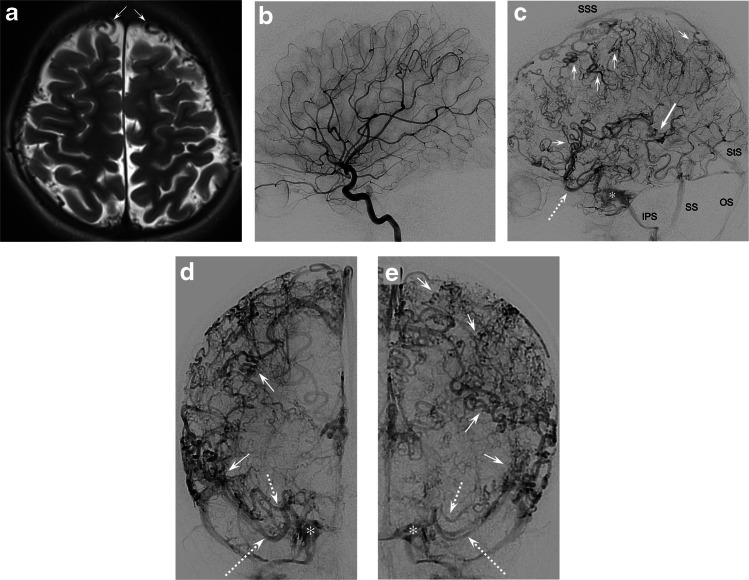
Non-restrictive cerebral venous dysgenesis in an 11-year-old girl with a history of infantile autism, attention deficit disorder, dyslexia, and camptodactyly. a Axial unenhanced T2-weighted image shows multiple small tortuous veins in the subarachnoid space above the cerebral hemispheres (*arrows*). b 2-dimensional (2-D) digital subtraction angiography (DSA), right internal carotid artery (ICA), mid-arterial phase, lateral: Normal appearance with no signs of arteriovenous shunting. c 2-D DSA, right ICA, mid-venous phase, lateral: filling of small superficial cortical veins with marked tortuosities and coilings over the right fronto-parietal and temporal cerebral hemisphere and in the Sylvian territory (*small arrows*). Drainage into the *SSS* and *SS*, and towards the cavernous sinus (CS, *asterisk*) and *IPS* via Sylvian veins (*broken arrow*). Deep venous drainage via tortuous ICV (*long arrow*) into the *StS* and *OS*. d, e 2-D DSA, right (d) and left (e) ICA, mid-venous phase, anteroposterior: extensive tortuosities and coilings of superficial pial and small cortical veins (*small arrows*). Bilateral superficial (*long broken arrow*) and deep (*short broken arrow*) Sylvian veins drain into the CS (*asterisk*). *ICV* internal cerebral vein, *IPS* inferior petrosal sinus, *OS* occipital sinus, *SS* sigmoid sinus, *SSS* superior sagittal sinus, *StS* straight sinus
